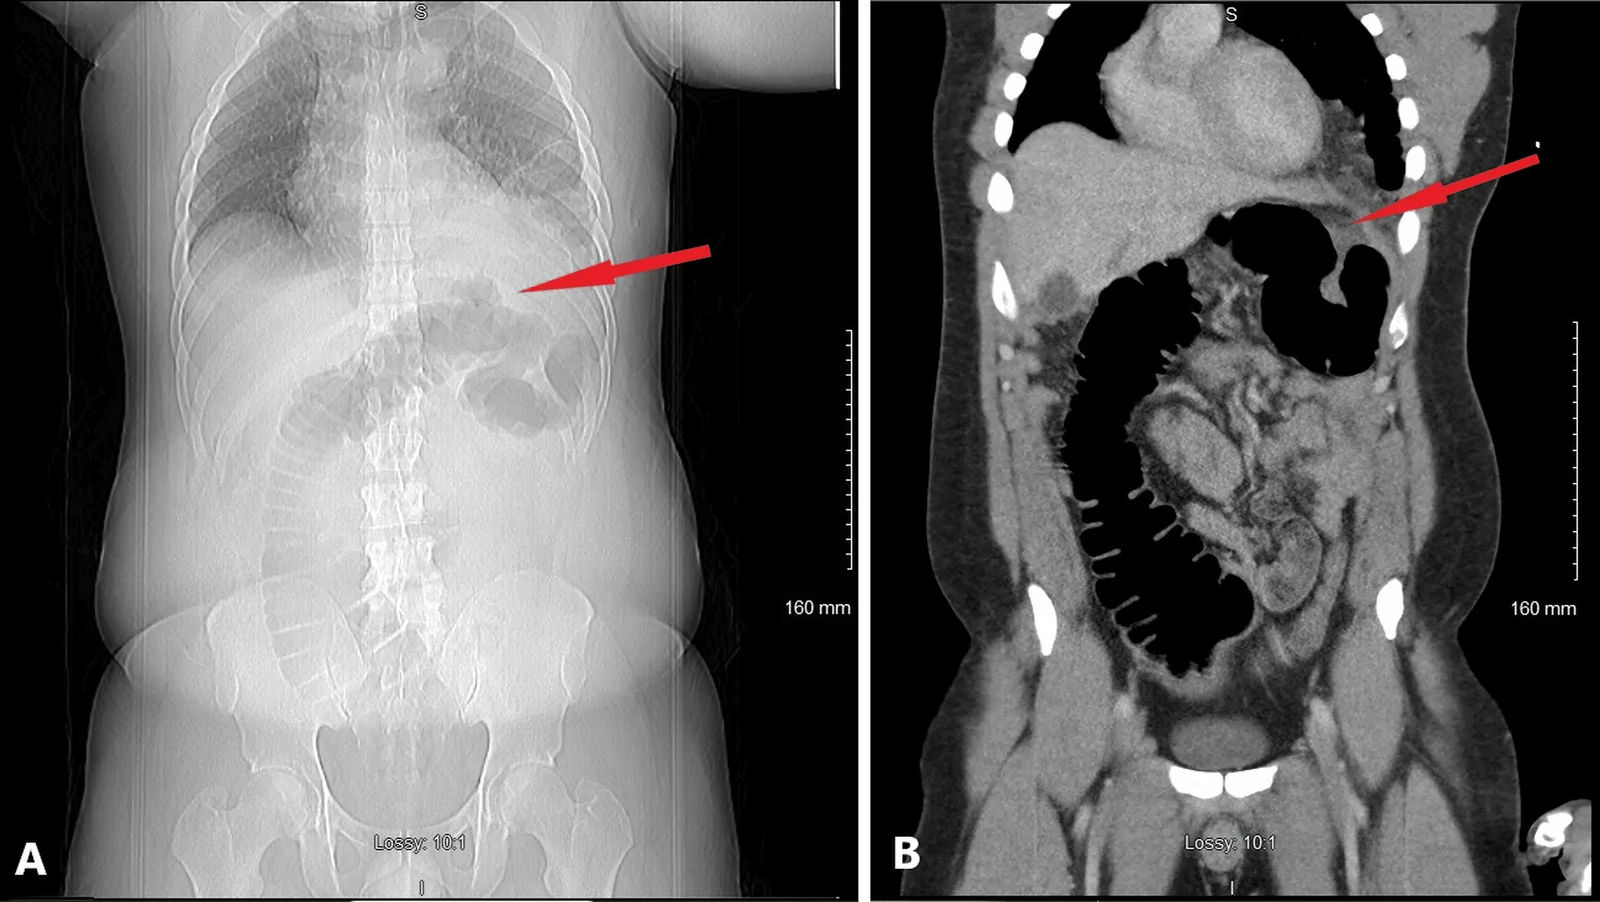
Preoperative noncontrast computed tomography. (A) Computed tomography scout image demonstrates gas-containing bowel loops projecting over the left lower hemithorax (red arrow), suggesting transdiaphragmatic herniation. (B) Coronal reconstruction demonstrates a left-sided posterolateral diaphragmatic (Bochdalek) defect (red arrow), with herniation of intra-abdominal contents, including bowel loops and omental tissue, into the left hemithorax.
